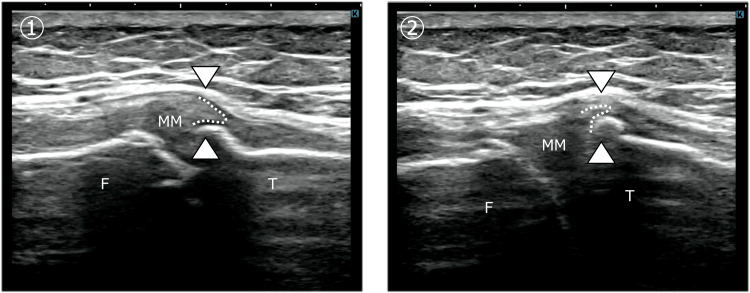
Restricted medial meniscus mobility due to osteophyte impingement in Case 2 Ultrasonographic images of the affected knee in Case 2 obtained at a slightly flexed position, demonstrating restricted medial meniscus (MM) dynamics during tibial rotation. Panel ① shows tibial internal rotation, and panel ② shows tibial external rotation. In both conditions, the tibial edge of the medial meniscus was impinged by a medial tibial osteophyte (arrowheads), preventing smooth excursion into and out of the joint space. This mechanical interference corresponded to the reproduction of movement-related pain. F: femur; T: tibia; MM: medial meniscus
